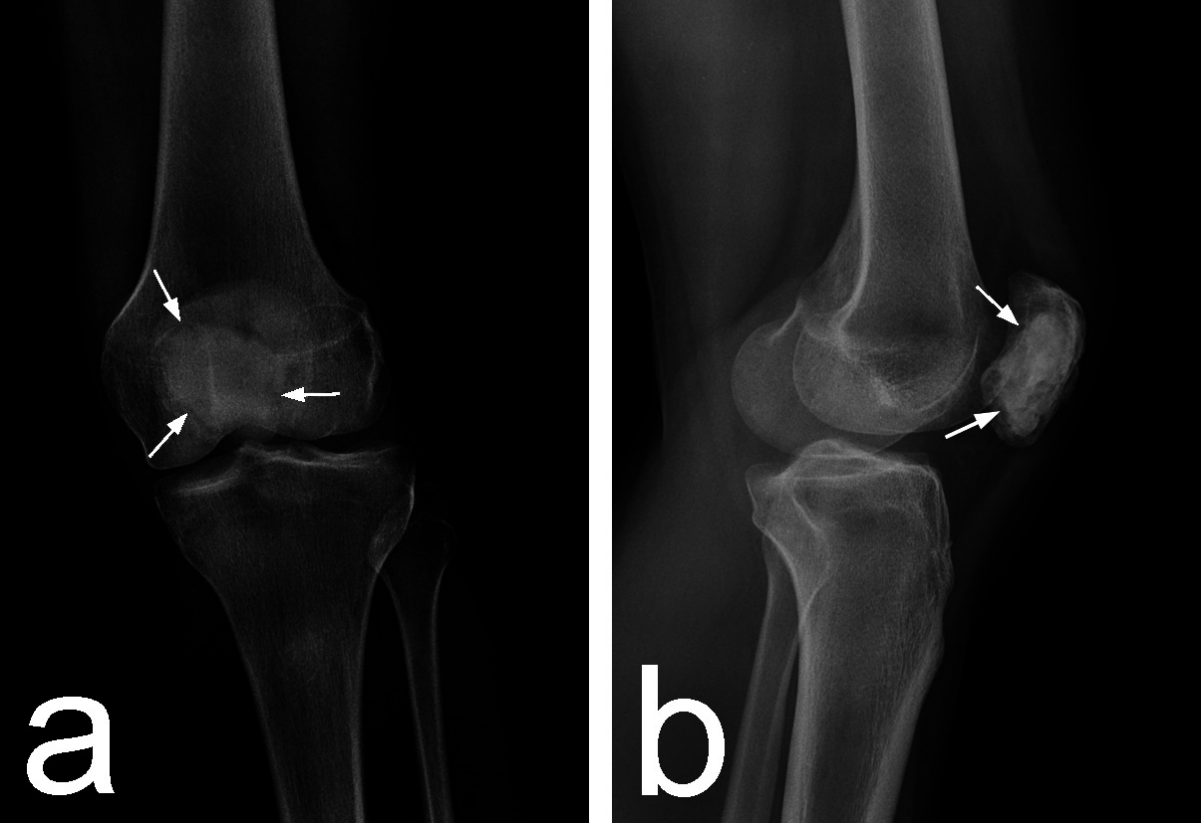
Plain radiographs at eight month follow-up A) AP and B) lateral radiographs of the knee showing "blurring" of the bone-TCP interface (arrows) as a sign of osteointegration of the graft. TCP: tricalcium phosphate
